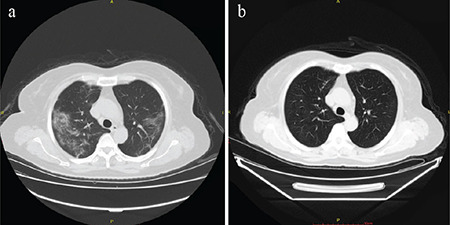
A 59-year-old man presented to the hospital because of close contact with a patient with COVID-19. Chest CT at admission shows multiple ground-glass shadows with interlobular septal thickening (a). After 14 days of isolation, chest CT results were normal (b). The patient recovered and was then discharged from the hospital. CT: computed tomography
